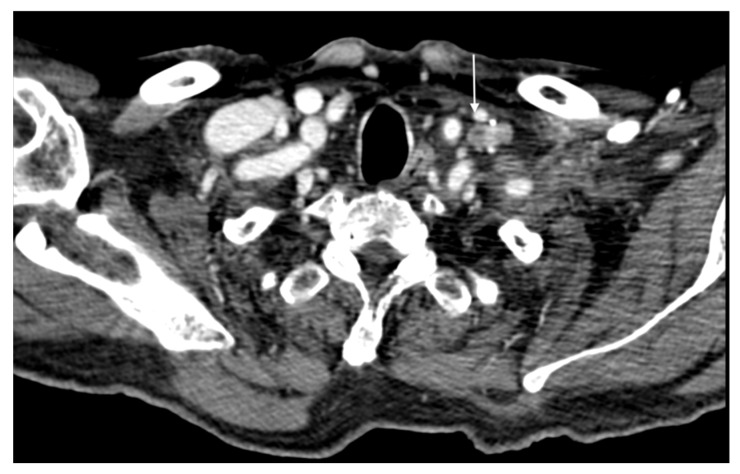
A 68-year-old man with known squamous cell carcinoma of the helix of the left auricle. Contrast-enhanced CT scan shows a positive latero-cervical lymph node. suspicious for metastatic involvement (white arrow). (Dual Source CT, Siemens Somatom^®^, Siemens Healthcare, Forcheim, Germany).
